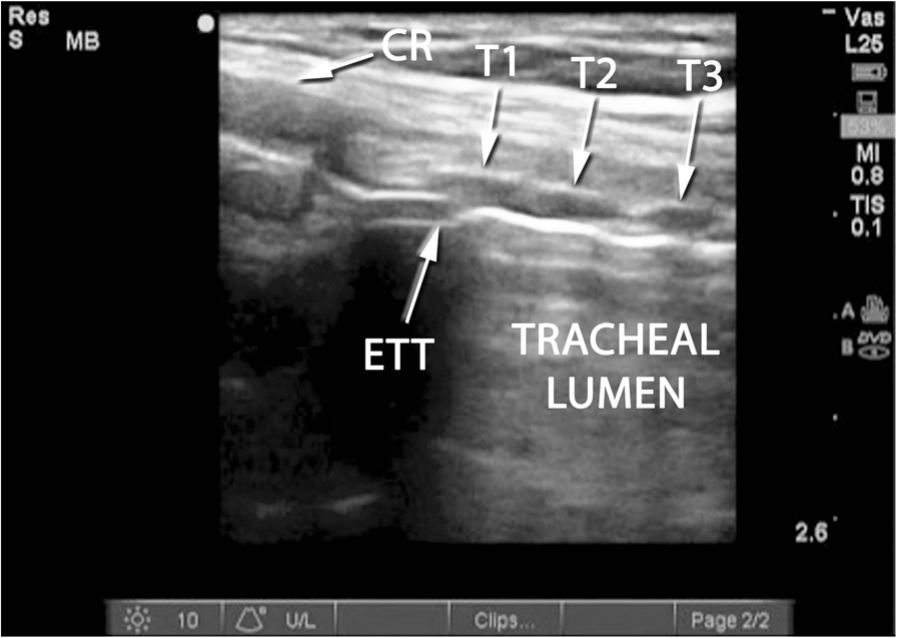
Endotracheal tube position on ultrasound. Longitudinal view of the trachea, demonstrating final positioning of the endotracheal tube (ETT) tip prior to tracheal puncture. CR, cricoid cartilage in cross section; T1, first tracheal ring in cross section; T2, second tracheal ring in cross section; T3, third tracheal ring in cross section.
